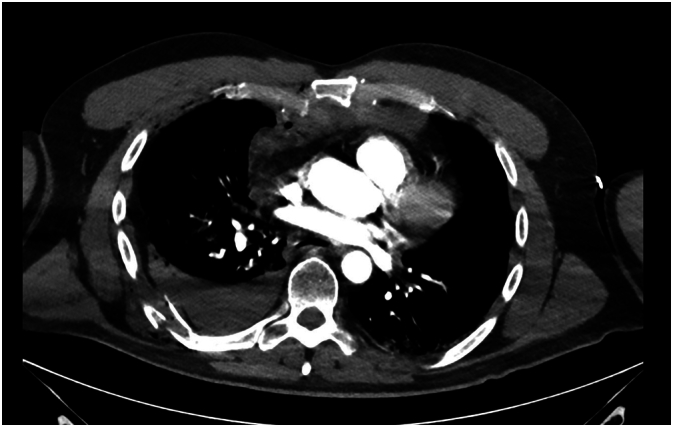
Computed tomography highlighting the costochondral separation of the right fourth intercostal space and right hemothorax.
